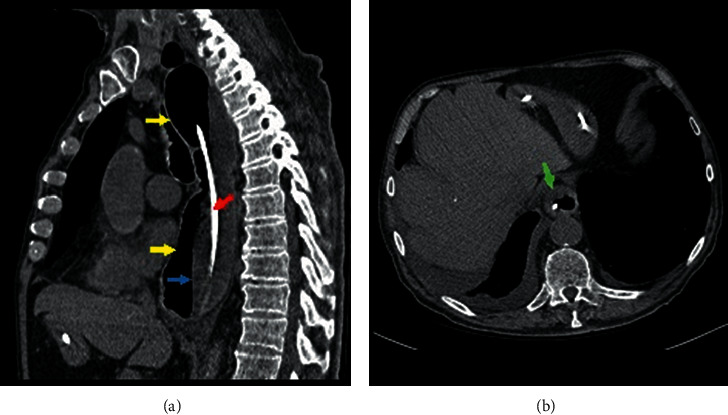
Computed tomography of the chest without IV contrast. Both sagittal (a) and axial (b) images demonstrating esophageal dilatation (yellow arrows) with an air-fluid level (blue arrow). There is a region of esophageal wall thickening near the gastroesophageal junction (green arrow) and an enteric tube (red arrow) is also noted.
